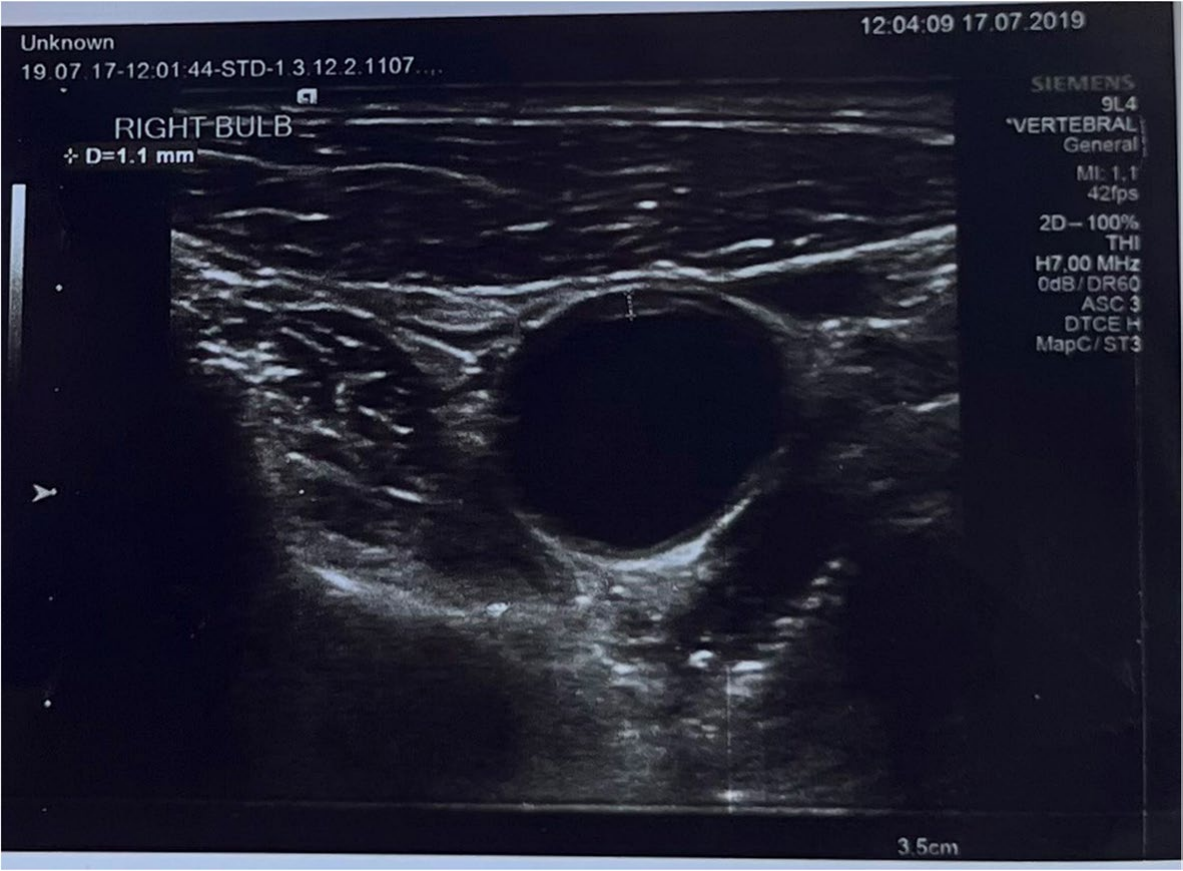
Measurement of right bulb of common carotid artery in COPD diagnosed patient by ultrasound
